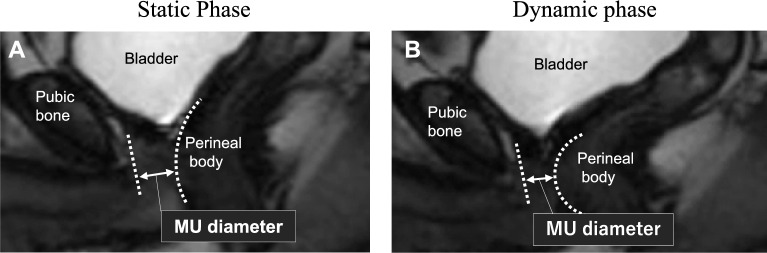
Antero-posterior diameter of the membranous urethra (MU diameter) in static (A) and dynamic phases (B) during the Valsalva maneuver by cine MRI.
